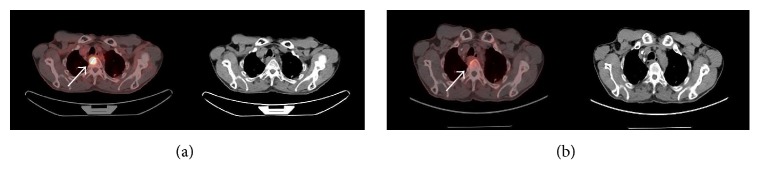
^18^F-FDG (a) and ^18^F-Alfatide II (b) PET/CT images of a thoracic tuberculosis patient. T2, T3, and T4 showed intense ^18^F-FDG uptake and mild ^18^F-Alfatide II uptake. The white arrows indicate tuberculosis lesions in T4.
